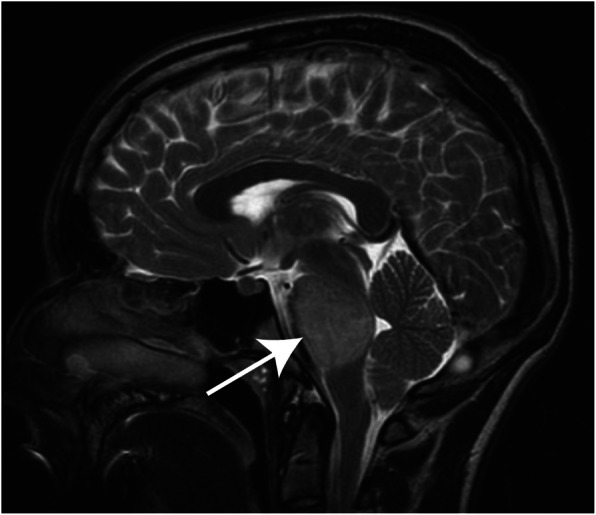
T2-weighted sagittal pediatric brain MRI. Characteristic diagnostic T2-weighted MRI of pediatric DIPG. Note brainstem which demonstrates a diffuse expansile hyperintense lesion in the pons (arrow). These tumors are surgically unresectable due to their poorly circumscribed border and highly eloquent location. Where safe-entry zones are obeyed and neuromonitoring is employed, incisional biopsies under direct observation can be performed
